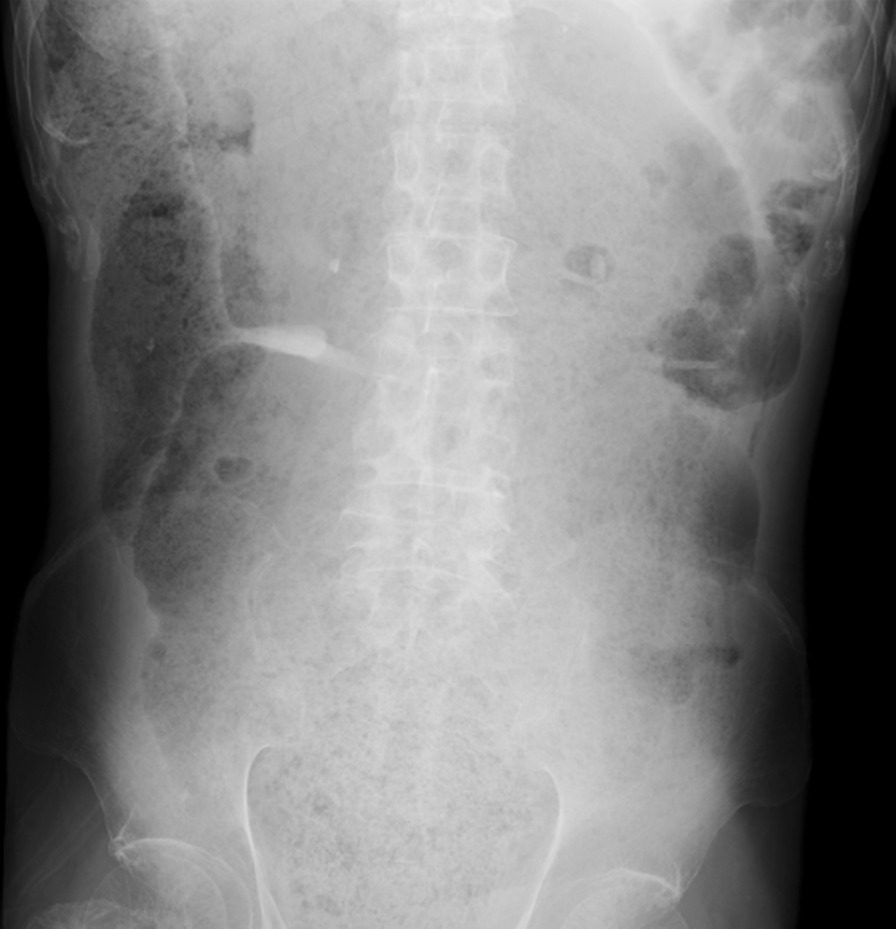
An abdominal X-ray on admission revealed a thickened gut wall and extremely dilated sigmoid colon and rectum filled with a massive amount of stool as well as gas
